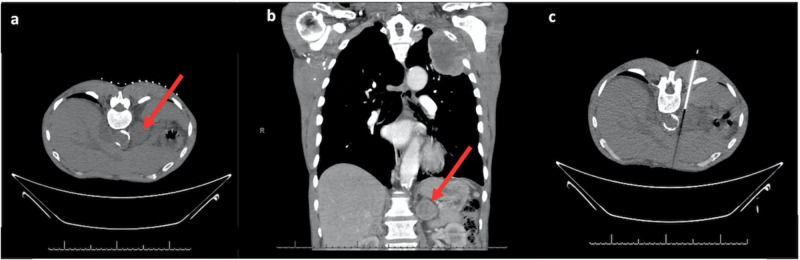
CT images of the chest showing heterogeneous enlarged left adrenal mass concerning for metastatic lesion Axial (a) and coronal (b) CT images of the chest demonstrating a heterogeneous enlarged left adrenal mass concerning for metastatic lesion (arrow). (c) Axial image obtained during CT-guided biopsy for tissue sampling.
